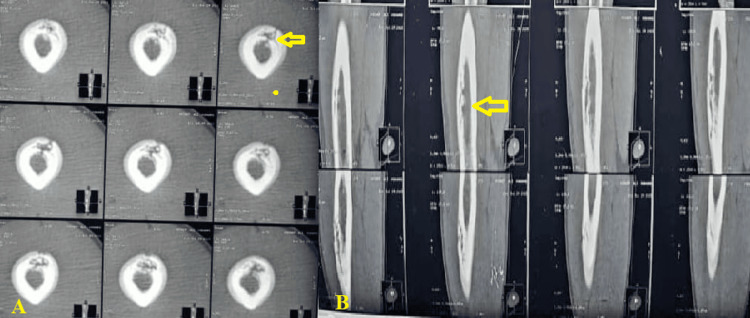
CT of the left femur Coronal reformatted image (A) and axial slice (B) at the level of the mid-diaphysis. The images reveal a well-defined sequestrum (a fragment of dense, necrotic bone) located within a prominent cloaca or drainage tract (yellow arrows). There is evidence of extensive circumferential periosteal reaction and involucrum formation (a layer of new bone growth surrounding the infected area), resulting in significant cortical thickening and narrowing of the medullary canal. These combined findings are characteristic of chronic osteomyelitis. CT: computed tomography
